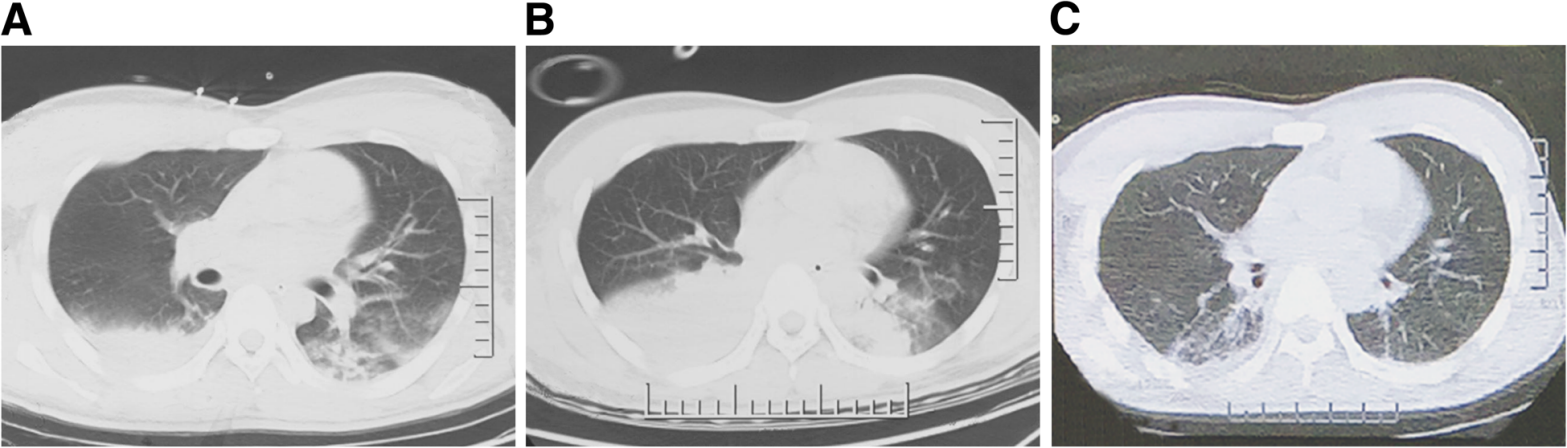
A The chest CT scan obtained 3 days post trauma. B The chest CT scan obtained 8 days post trauma. C The chest CT scan obtained 22 days post trauma (14 days post anti-sepsis bundles)
